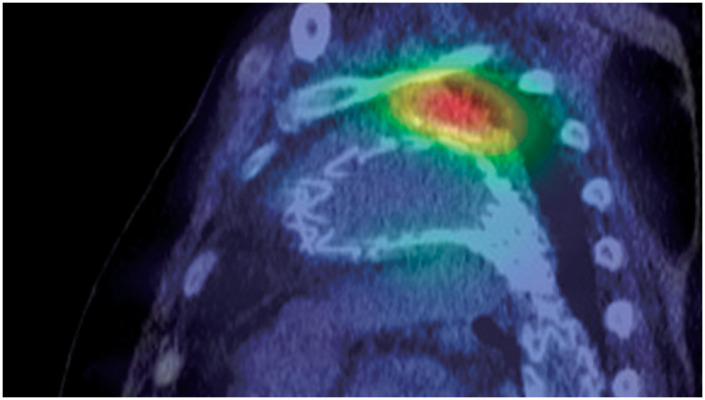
Preoperative gallium scintigraphy findings indicating the presence of an infected thoracic aortic aneurysm and stent-graft infection.
